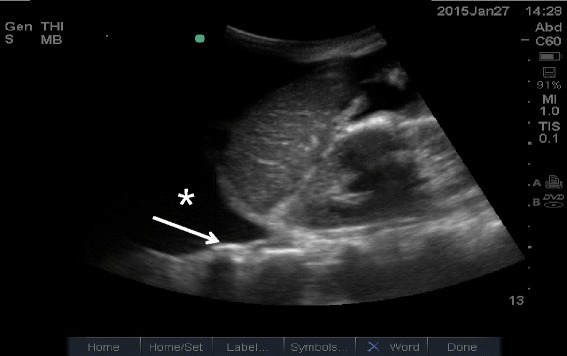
Pleural effusion. Pleural effusion (*asterisk*) permits the ultrasound beam to penetrate deeply to reveal the vertebral stripe (*arrow*). The vertebral stripe will not be visible above the diaphragm if the lung is aerated
